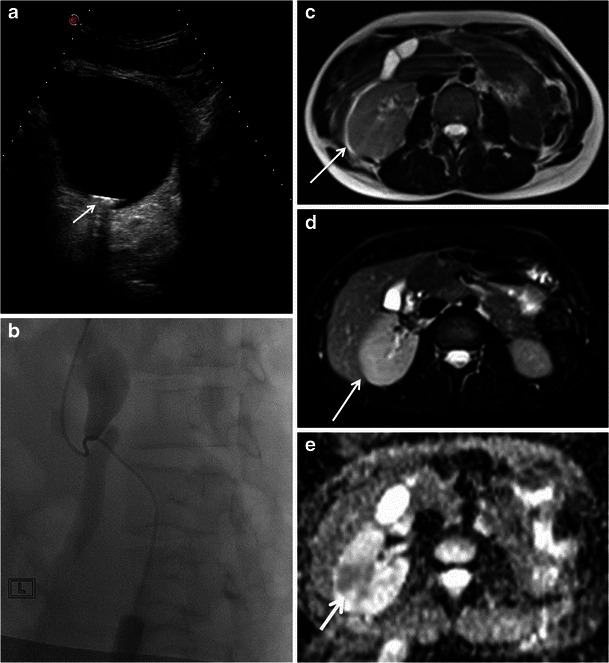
A 28-year-old woman was admitted at 35 weeks of gestation presenting renal colic not responding to medical treatment. US images showed left hydronephrosis due to a 1-cm stone impacted at the level of the ureterovesical junction (a). A percutaneous nephrostomy was performed and the stone extracted (b). Two days after this procedure, the patient presented with fever and acute pain localized to the left flank. Ultrasound was unremarkable. The axial T2-weighted HASTE sequence (c) and axial T2-weighted fat-saturated sequence (d) show an enlarged edematous right kidney; focal areas of higher signal intensity on T2-weighted imaging could be due to focal infection. ADC map (e) shows restricted proton diffusion indicative of pyelonephritis
